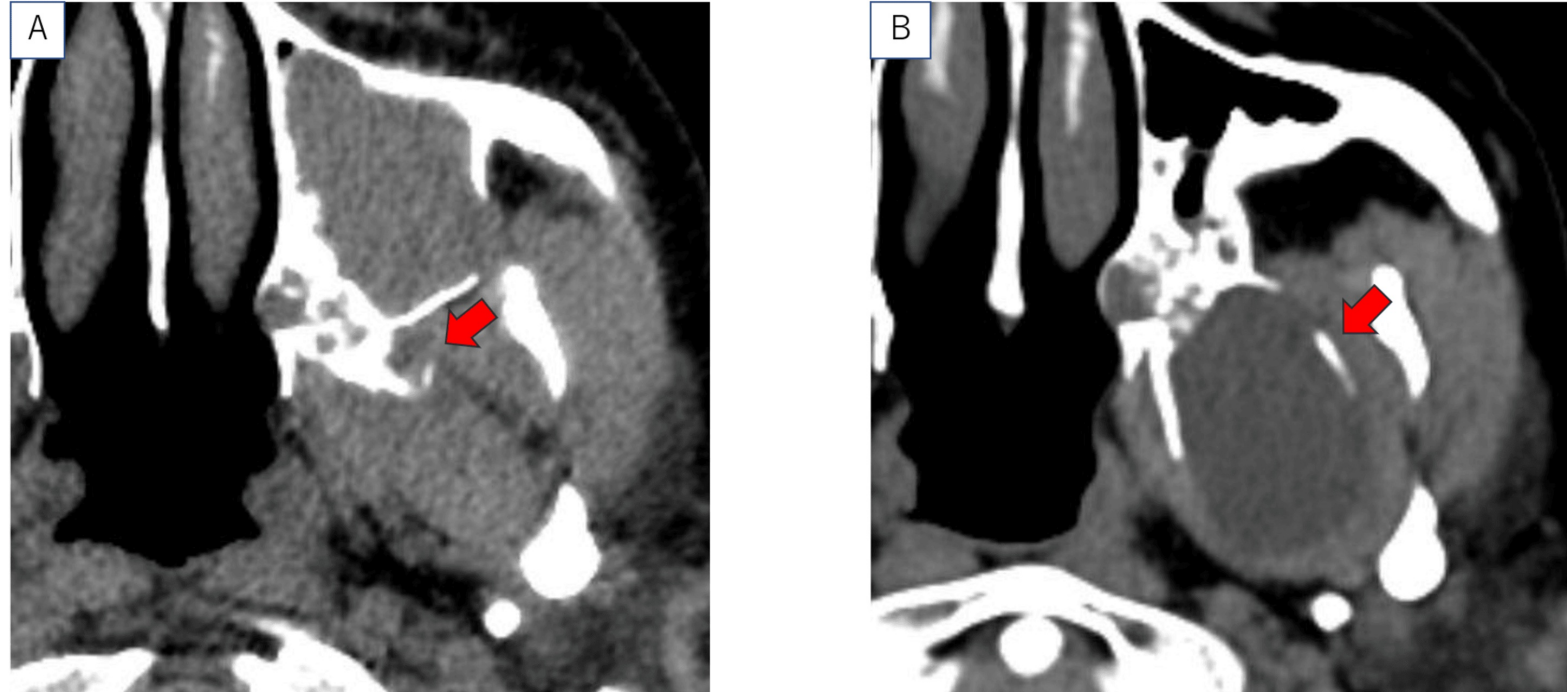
Horizontal cross-sectional CT image. Horizontal cross-sectional CT image taken at another hospital four years ago (A) and at our hospital during the first consultation (B). The red arrow indicates the lesion.
